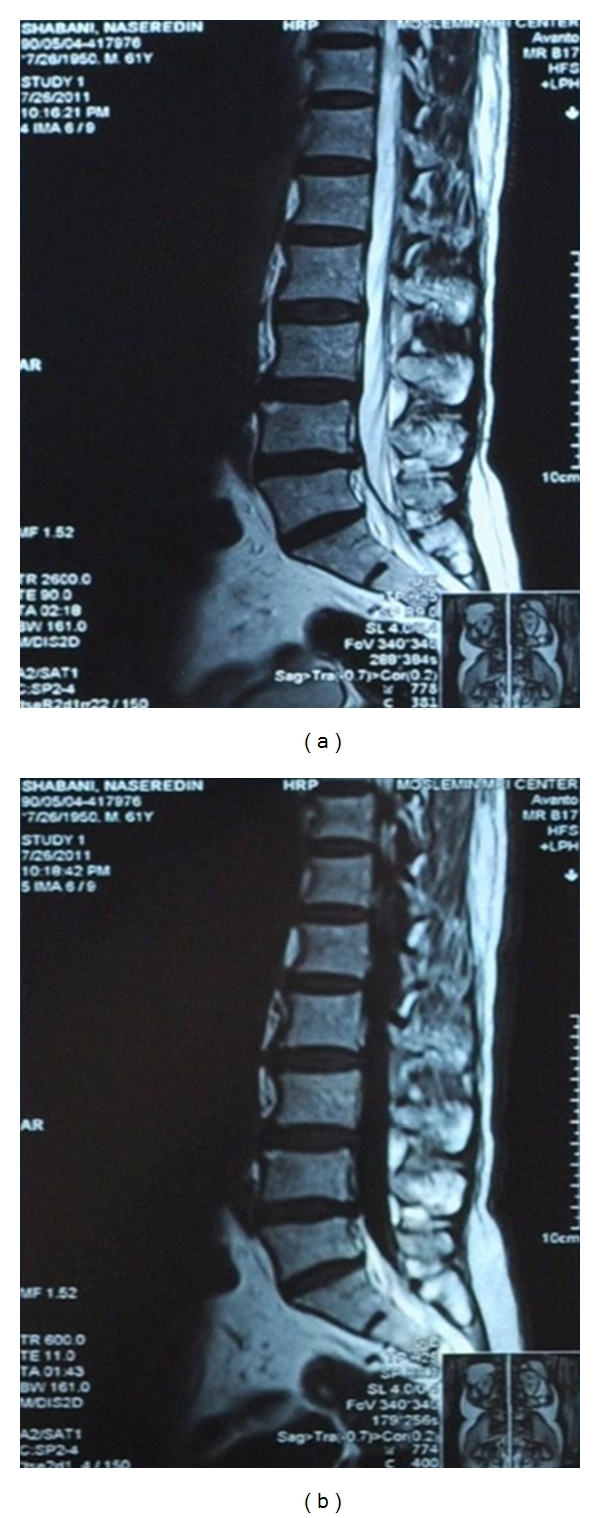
On sagittal T1-weighted (a) and T2-weighted (b) lumbar MR images lumbar degenerative joint change and lumbar disc dehydration with decreased lumbar lordosis is seen.
